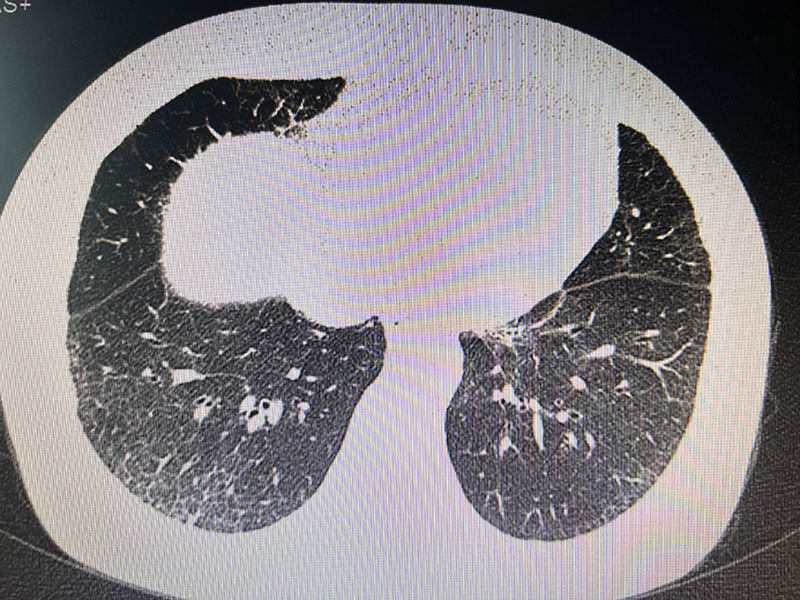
Computed tomography found some interstitial changes in the lower lobes of both lungs.
